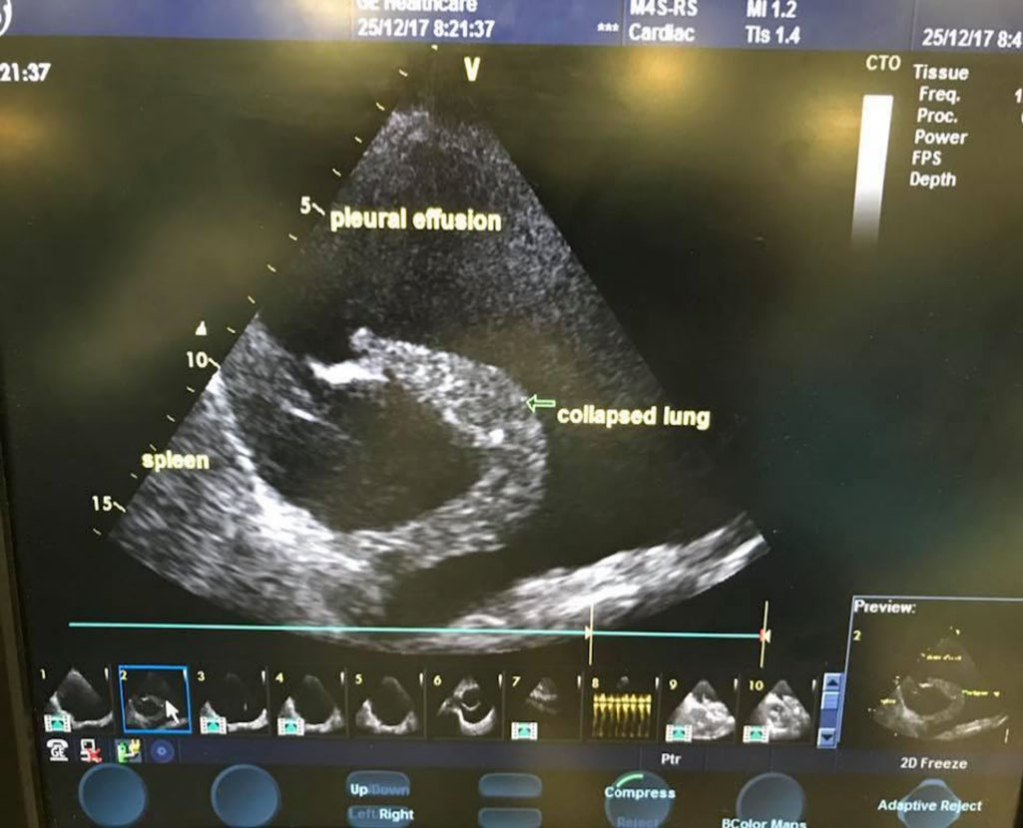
ultrasound chest shows Left pleural effusion. This scan shows substantial effusion with multiple echoes, mobile, and whirling in real‐time (plankton sign). The lower lobe is consolidated
